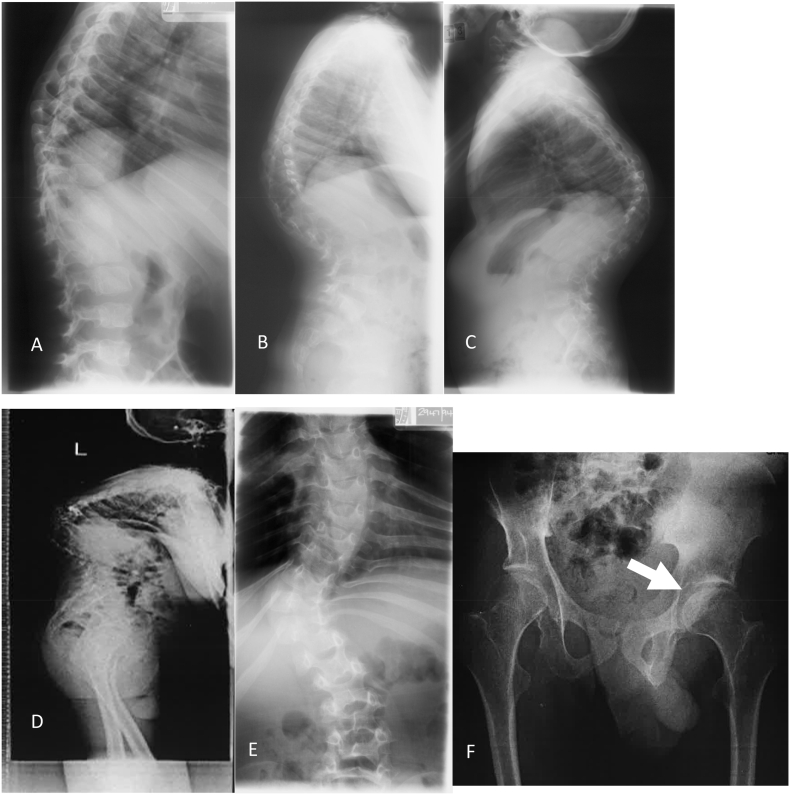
X-ray images of dysostosis multiplex showing progressive kyphosis at 4 years (A), 5 years (B), 7 years (C) and 28 years (D), significant scoliosis (E) and dysplastic hips (F), shallow hip sockets and femur head partly uncovered (arrow).
